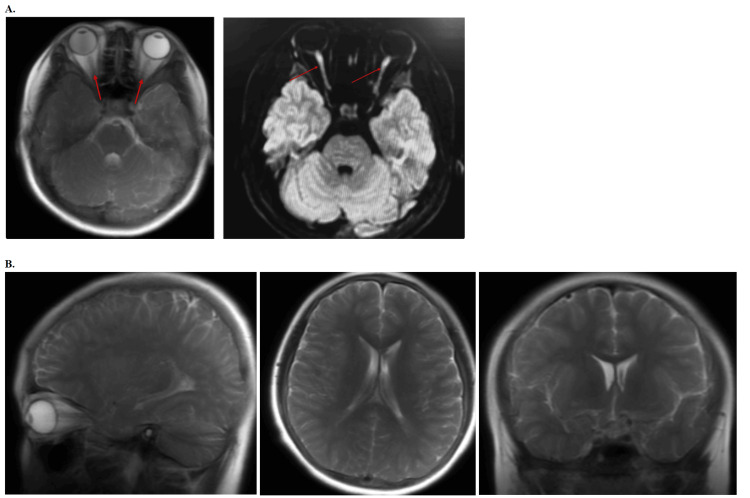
Gadolinium-enhanced T2-weighted MRI images demonstrating optic nerve and brain anatomy in a patient with a suspected neurological disorder A. Left panel shows the axial T2-weighted MRI using an FSE sequence, with gadolinium-based contrast agent enhancement, highlighting the optic nerves (indicated by red arrows). This sequence is particularly useful for detecting changes in the optic nerves and surrounding structures post-contrast administration. Right panel shows the axial T2-weighted MRI using a FLAIR sequence, post-gadolinium-based contrast, which enhances the visibility of lesions in the white matter by suppressing the cerebrospinal fluid signal. The red arrows indicate subtle areas of interest around the optic nerves. B. Left panel shows the sagittal T2-weighted MRI using an FSE sequence with gadolinium-based contrast agent enhancement, providing a detailed view of the brain’s midline structures. The T2-weighted images offer clear visualization of brain parenchyma and cerebrospinal fluid spaces, useful for identifying areas of edema or demyelination. Middle panel shows the axial T2-weighted MRI using an FSE sequence with gadolinium-based contrast agent, offering a clear depiction of the brain’s deep structures, including the ventricles and surrounding tissues. Right panel shows the coronal T2-weighted MRI using an FSE sequence with gadolinium-based contrast agent, showing a cross-sectional view of the brain and optic nerves. These T2-weighted images were obtained using a fat-suppressed sequence to enhance the visualization of the optic nerves and associated structures. FSE, fast spin echo; FLAIR, fluid-attenuated inversion recovery
